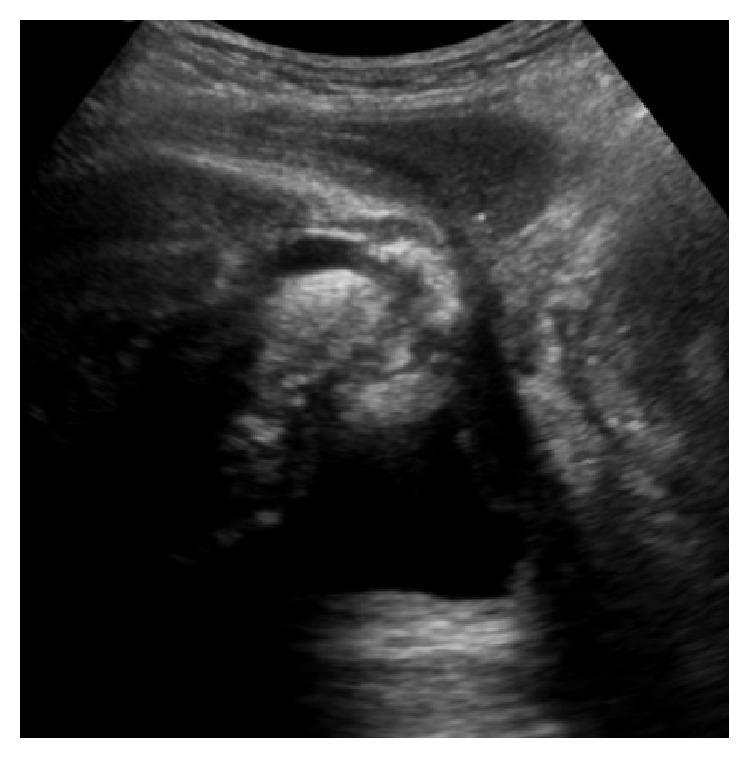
Urethral bulb after radical prostatectomy (no prostate) in patient suffering from urinary incontinence. Explanation: images show perineal skin and bulb top and bladder below (deep), ventrally on left and dorsally on right side as it appears on page; ultrasound probe oriented in midsagittal plane.
